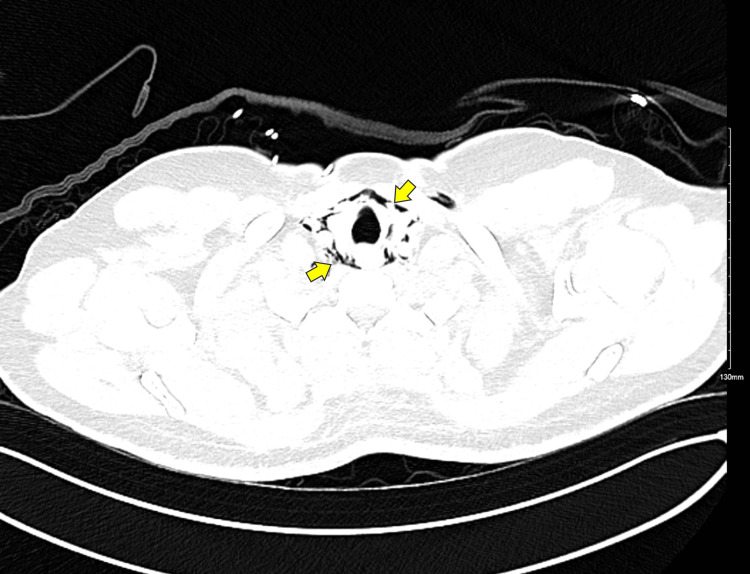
Axial chest CT with lung window highlighting pneumomediastinum (arrows) CT: computed tomography
